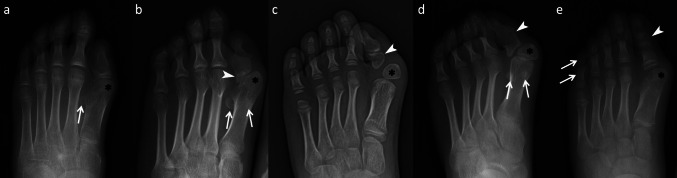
Radiographs of great toe malformations in fibrodysplasia ossificans progressiva (FOP) showing monophalangeal or biphalangeal hallux. a A 9-year-old female (Patient 10) with a monophalangeal great toe, mildly medially deviated malformed metatarsal head (asterisk), and hallucal sesamoids; the fibular sesamoid (arrowhead) is visible, whereas the tibial sesamoid is not. b A 20-year-old male (Patient 2) with mild hallux valgus and a monophalangeal great toe, showing a medially deviated malformed metatarsal head (asterisk), hallucal sesamoids (arrows), and an ectopic ossification center (EOC) (arrowhead). c A 6-year-old female (Patient 7) with a biphalangeal hallux and a proximal “delta phalanx” (arrowhead) with an asymmetric amorphous shape; an EOC (asterisk) is located at the distal-medial aspect of the hallux metatarsal head, with moderate hallux valgus. d Follow-up radiography at age 12 years of the same patient shown in c, demonstrating complete fusion of the first-digit phalanges (arrowhead), whereas the EOC (asterisk) persists; mild hallucal sesamoids are present. e A 9-year-old male (Patient 5) with a biphalangeal hallux and mild hallux valgus. Note the enlarged secondary ossification center (arrowhead) of the distal phalanx, the medially deviated malformed metatarsal head (asterisk), and a biphalangeal fifth digit (arrows)
